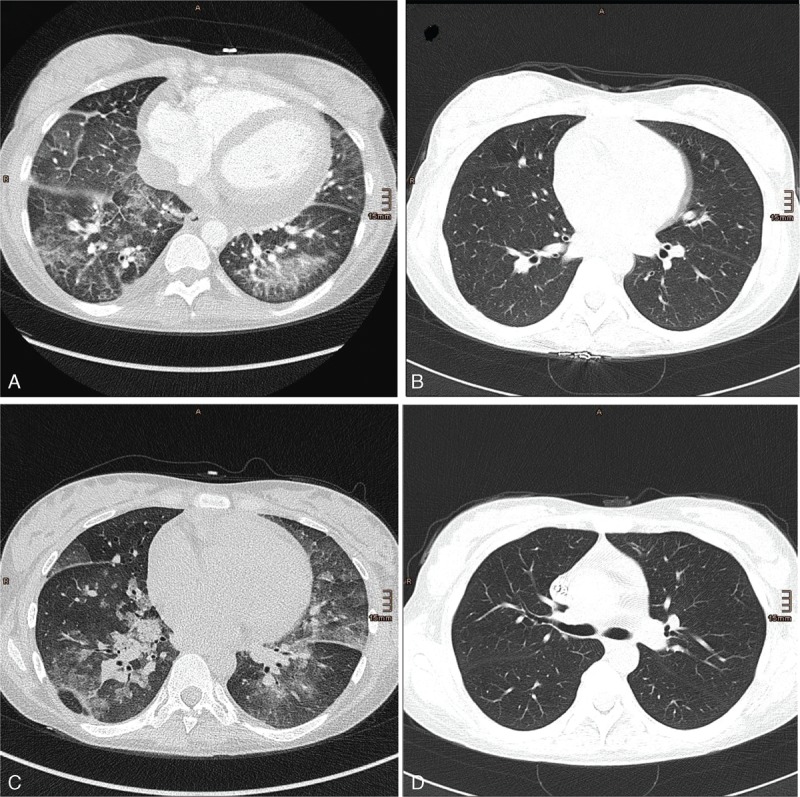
(A) Diffuse pulmonary hemorrhage in both lower lobes, which resolved after another initiation of (B) IAS. (C) After discontinuation of IAS, recurrence of pulmonary hemorrhage could be detected. These findings prompted us to initiate yet another series of IAS together with administration of eculizumab. (D) Complete resolution was detected in a control computed tomography 4 days later. IAS = immunoadsorption.
